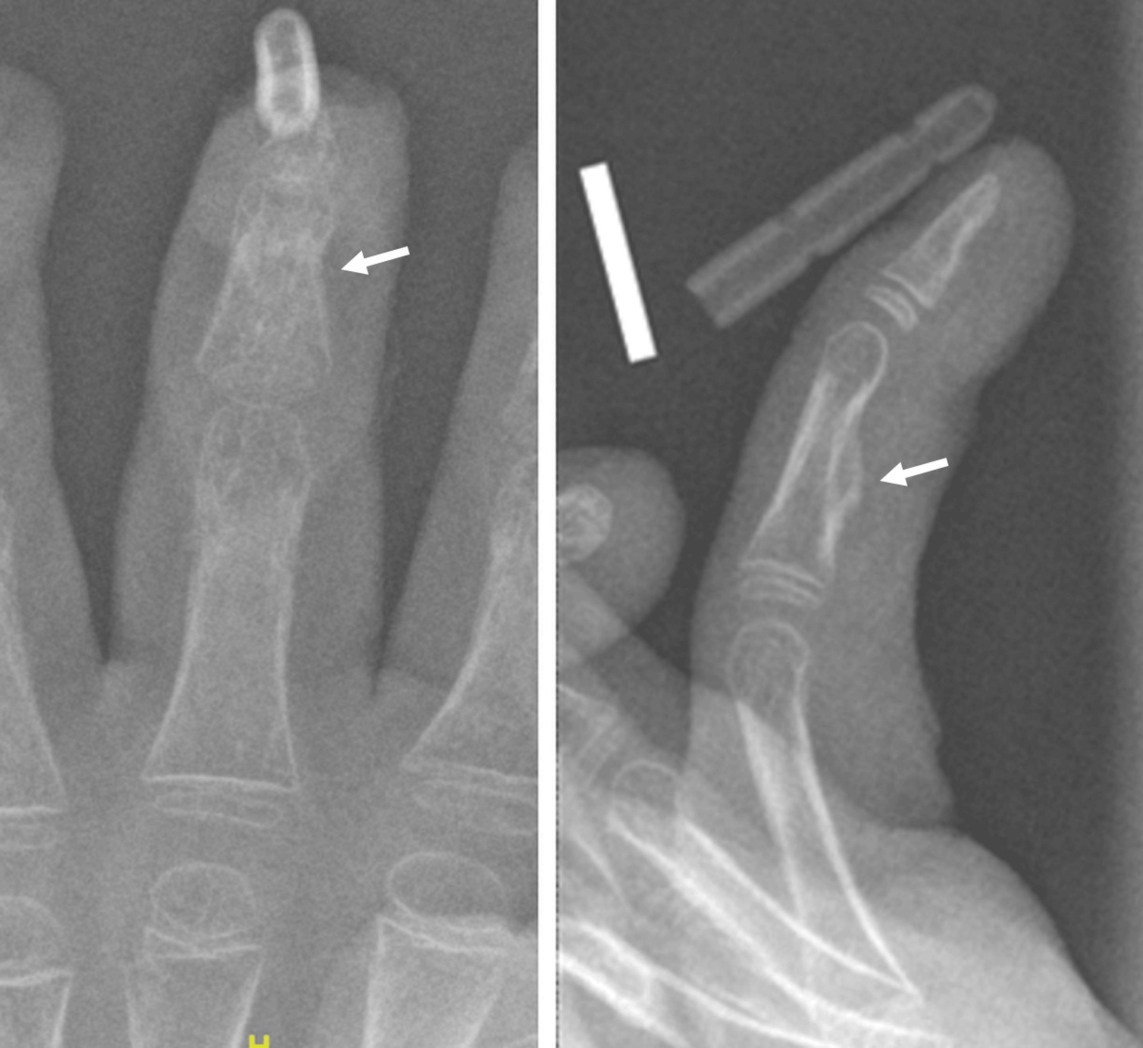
Consolidation of the initial ossification observed on follow-up radiographs obtained six weeks after surgery Left: anteroposterior view; Right: lateral view.
